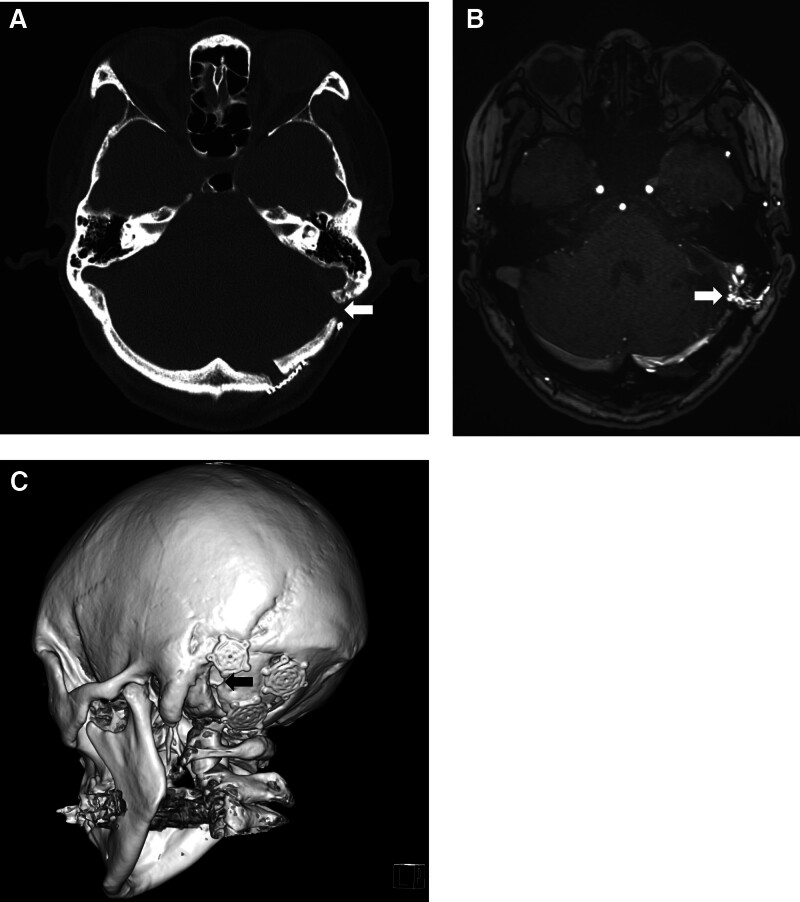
(A) Postoperative computerized tomography scan showing incomplete cranioplasty and remaining bone defect (arrow). (B) Noncontrast time-of-flight–magnetic resonance imaging showing that numerous branches of the occipital artery are directly connected to the sigmoid sinus through the bone defect (arrow). (C) Postoperative 3-dimensional reconstructive computerized tomography image showing incomplete cranioplasty (arrow indicates bone defect).
